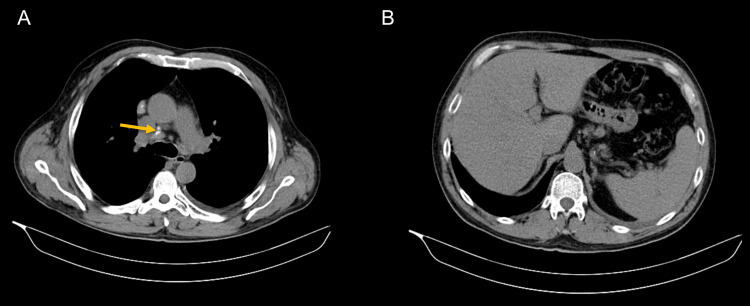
Thoraco-abdominal-pelvic CT scan: soft-tissue window (A) Partially calcified mediastinal lymphadenopathy (yellow arrow). (B) Diffuse hepatosplenomegaly.
